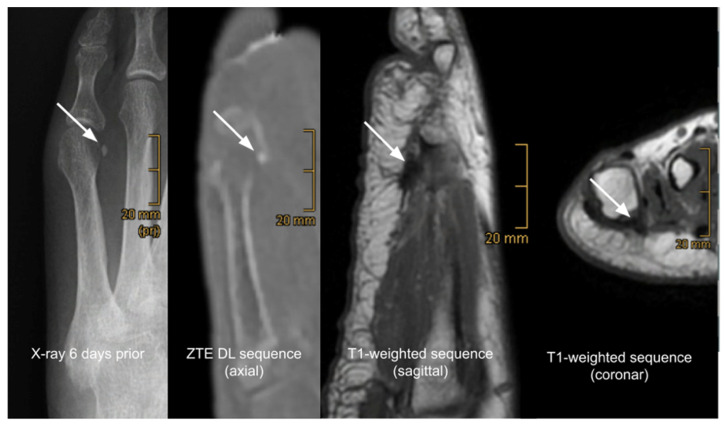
Seventy-three-year-old female with suspected Morton neuroma, in whom X-ray and MRI were performed, imaged with ZTE DL and coordination-matched sagittal and coronal T1-weighted MRI (no axial T1-weighted images were acquired), with a corresponding radiograph obtained 6 days earlier. An ossicle at the metatarsophalangeal joint V is visible on the radiograph and on ZTE DL, but not on the T1-weighted sequences.
